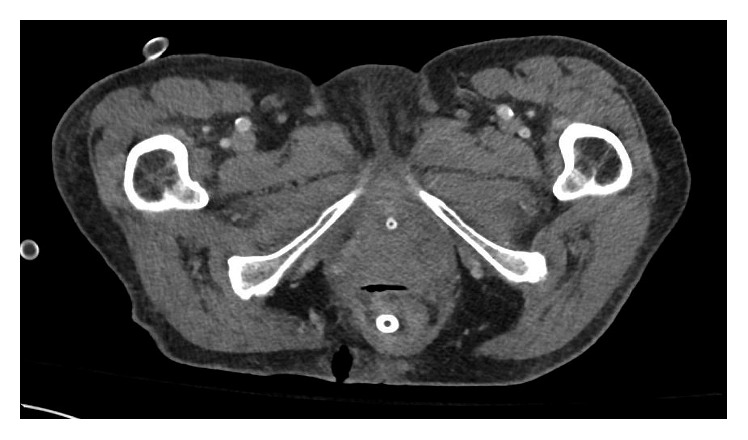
CT on day 7 after drainage demonstrating resolution of abscess with transanal drain in place.
